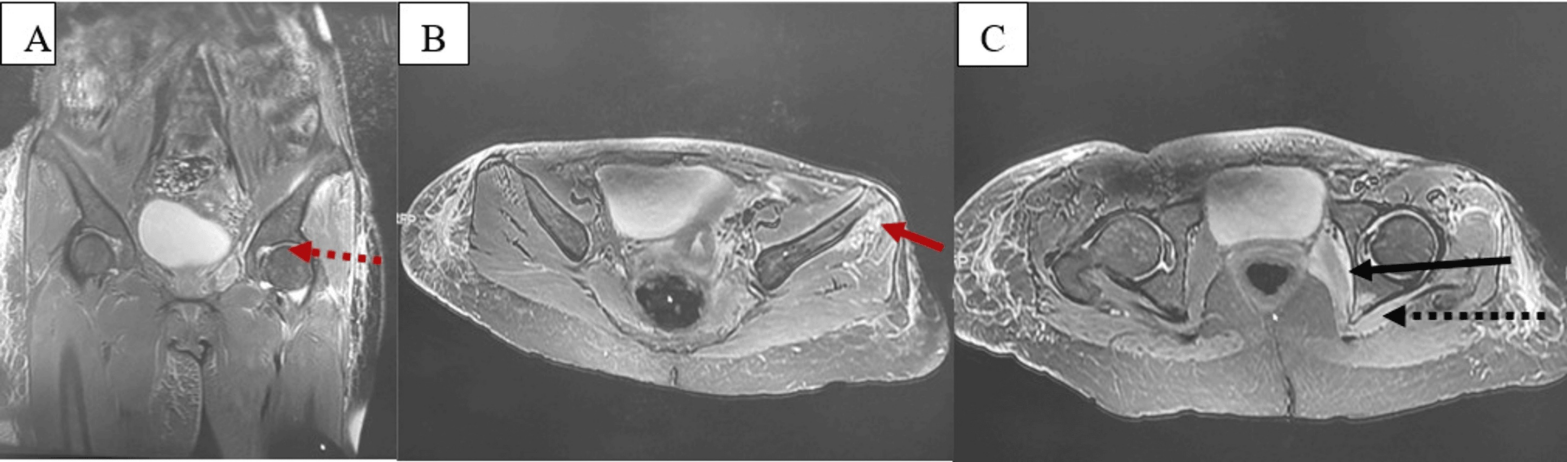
Pelvic MRI, T2-weighted sequences A: Left coxofemoral synovitis (red dotted arrow); B: Myositis of the left gluteus minimus (red solid arrow); C: Myositis of the left obturators (black solid arrow) and pelvitrochanteric muscles (black dotted arrow)
